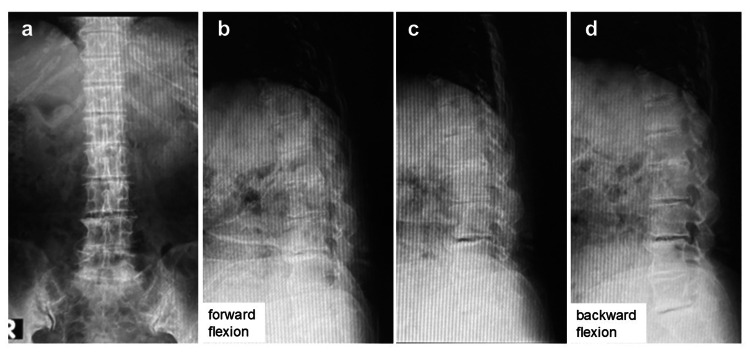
Preoperative X-ray Preoperative X-ray showing lumbar degeneration and mild kyphosis, not showing lumbar spondylolisthesis. (a) Anteroposterior view; (b) forward flexion; (c) neutral position; (d) backward flexion.
